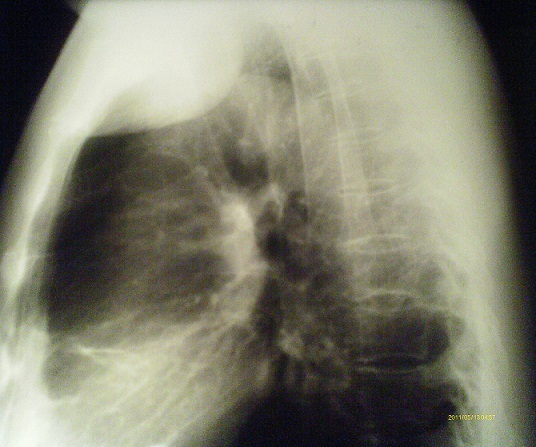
Radiographie du thorax en incidence de profil: Masse unique avec lyse costale massive et extension extra-pleurale et dans les parties molles
